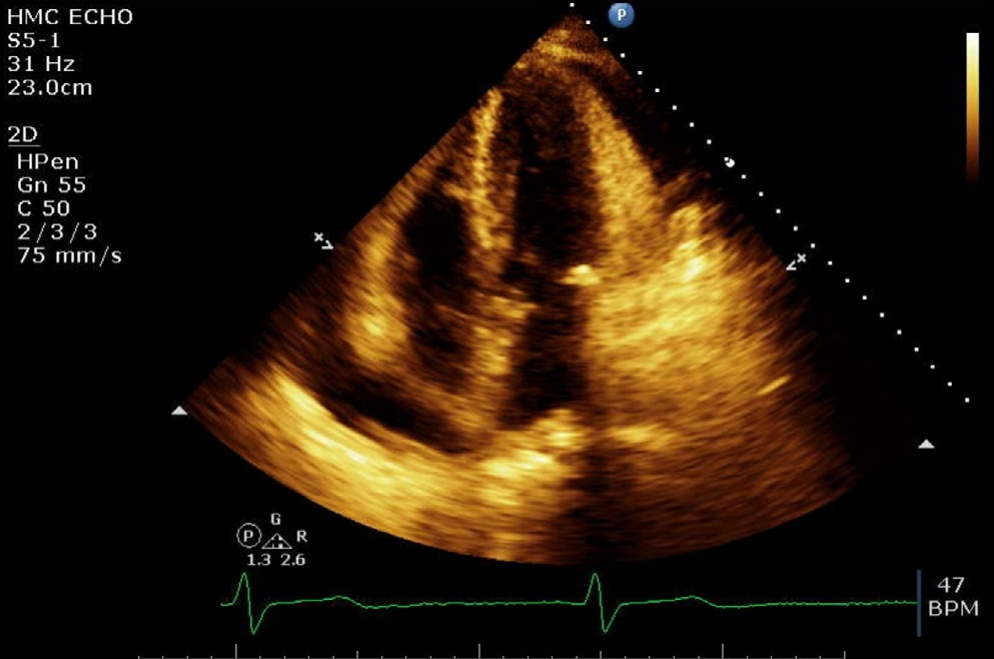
Transthoracic echocardiogram with four‐chamber view showing pericardial effusion
